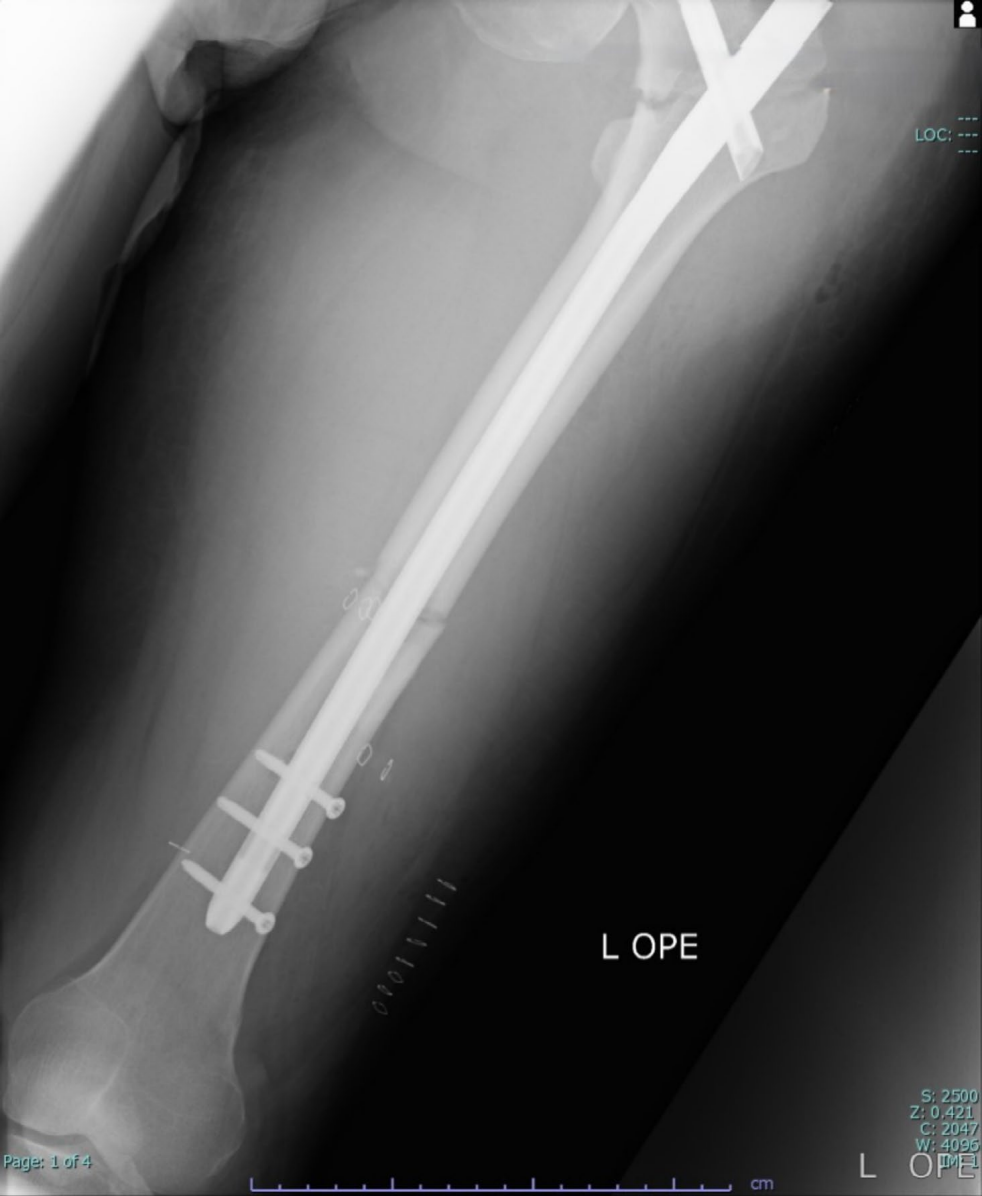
Postoperative plain radiograph demonstrating fixation of the left femoral shaft and trochanteric fractures.
